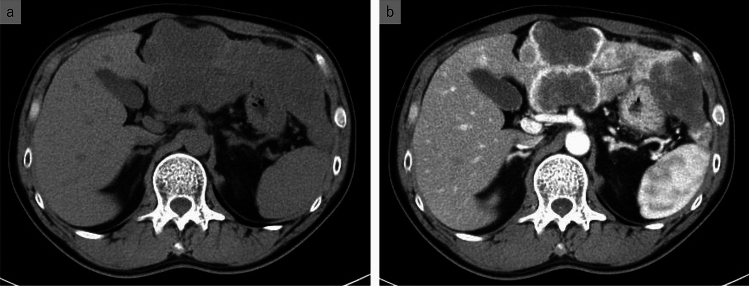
Radiologic findings of a primary hepatic alpha-fetoprotein-producing neuroendocrine neoplasm. a Pre-contrast CT image reveals multiple tumorous lesions within the liver. b Contrast-enhanced arterial phase of the CT scan. Strong contrast enhancement is evident at the periphery of the occupying lesion, while the interior shows hypovascularity. In addition, the tumor periphery exposed to the liver surface exhibits a concave indentation. These findings are consistent with those of intrahepatic cholangiocarcinoma. *CT* computed tomography
